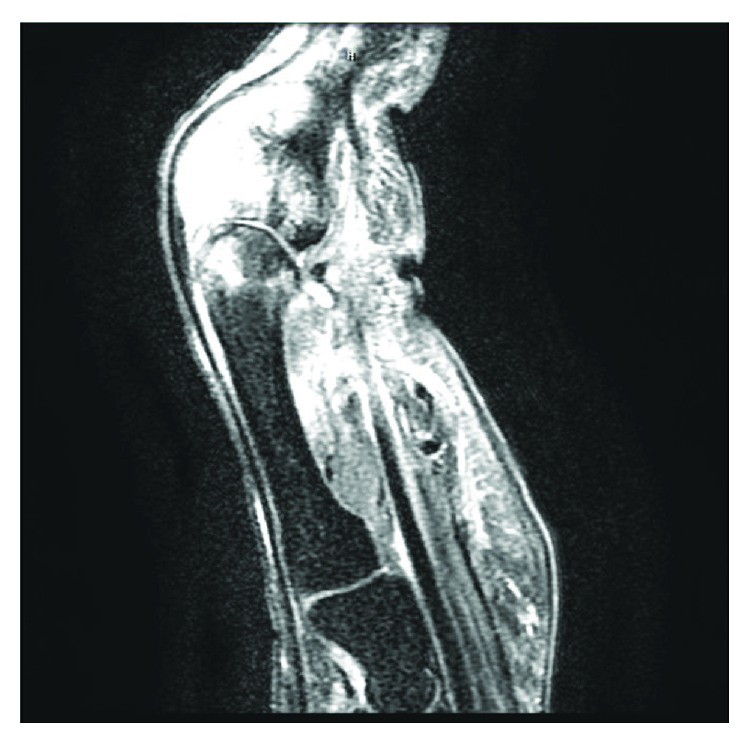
T2 sagittal MRI image of the described chondrosarcoma.
